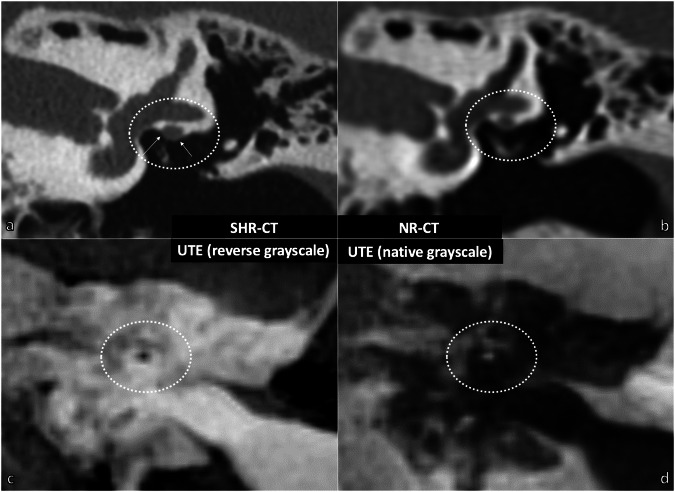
Osseous coverage of the tympanic portion of the facial nerve analyzed with CT and UTE-MRI sequences in the coronal plane (example in living patient). a SHR-CT and (b) NR-CT showing a clearly visible osseous coverage (white arrows), whereas it is indistinguishable in the UTE-MRI sequence with inverted grayscale (c) and native grayscale (d), as well as the air-bone interface in the dotted circle. CT*,* Computed tomography; MRI, Magnetic resonance imaging; NR*,* Normal resolution; SHR*,* Super-high resolution; UTE, Ultrashort time of echo
